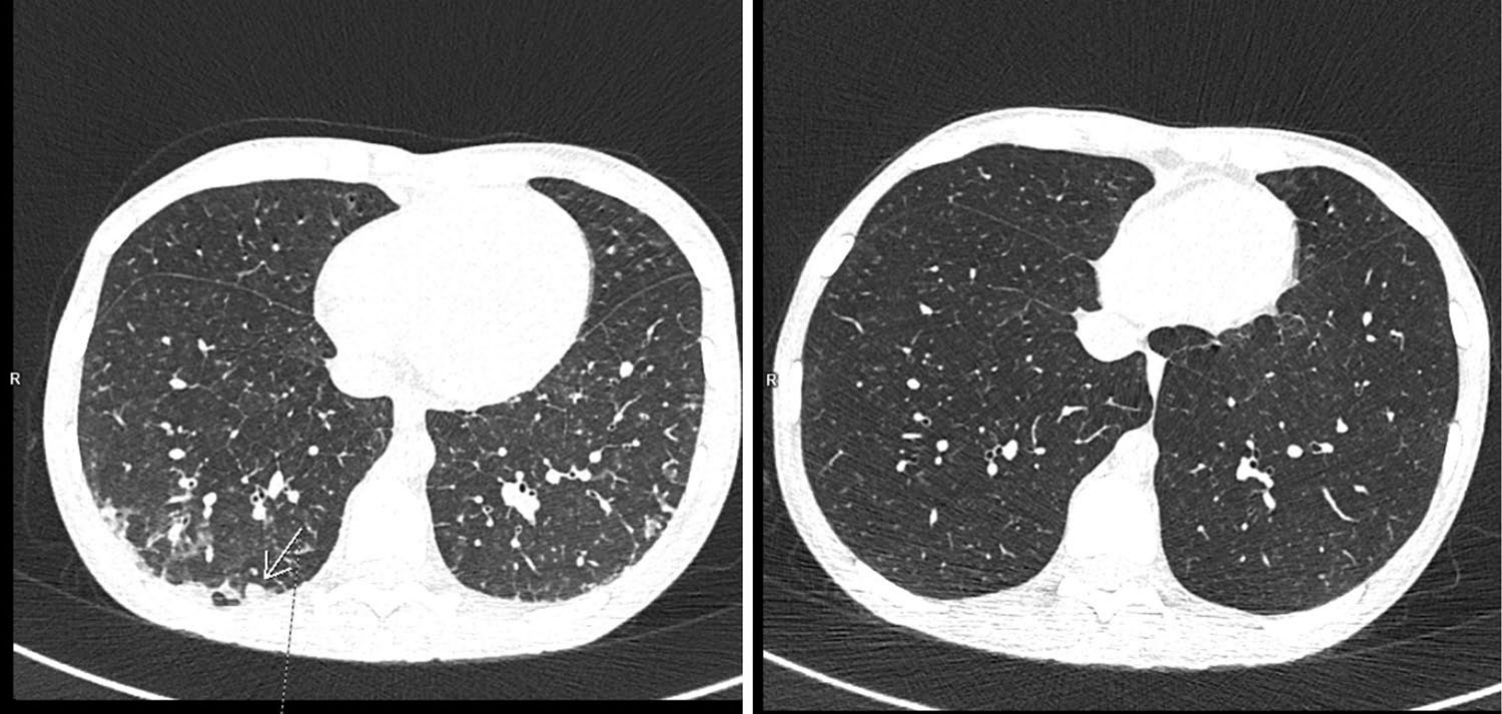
Left side: First CT scan. Numerous intralobular small nodules disseminated bilaterally, white arrow—one of them located near the thoracic wall. Right side: Second CT scan, the same area after treatment
